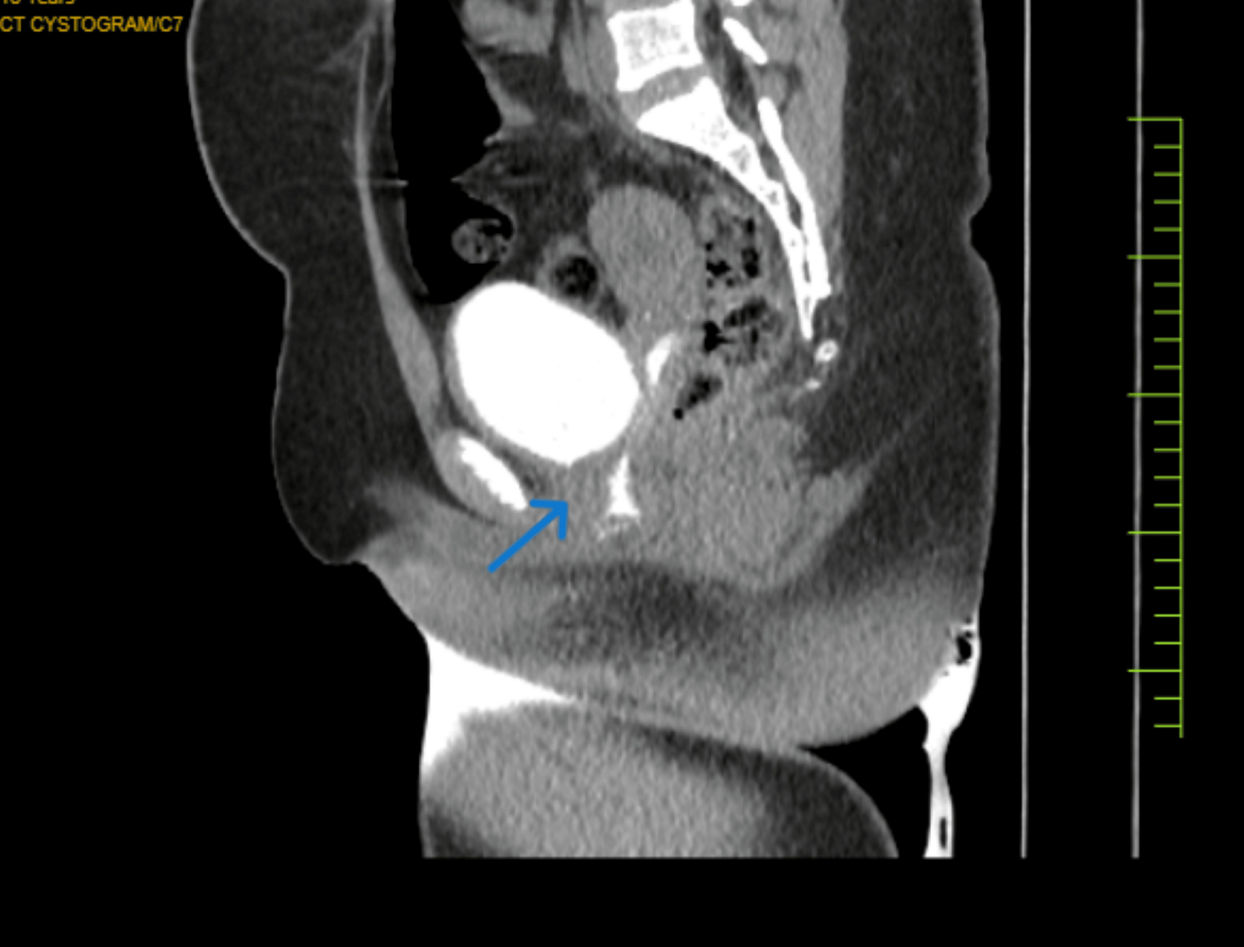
CT cystography Reflux of contrast from urinary bladder into the vagina
